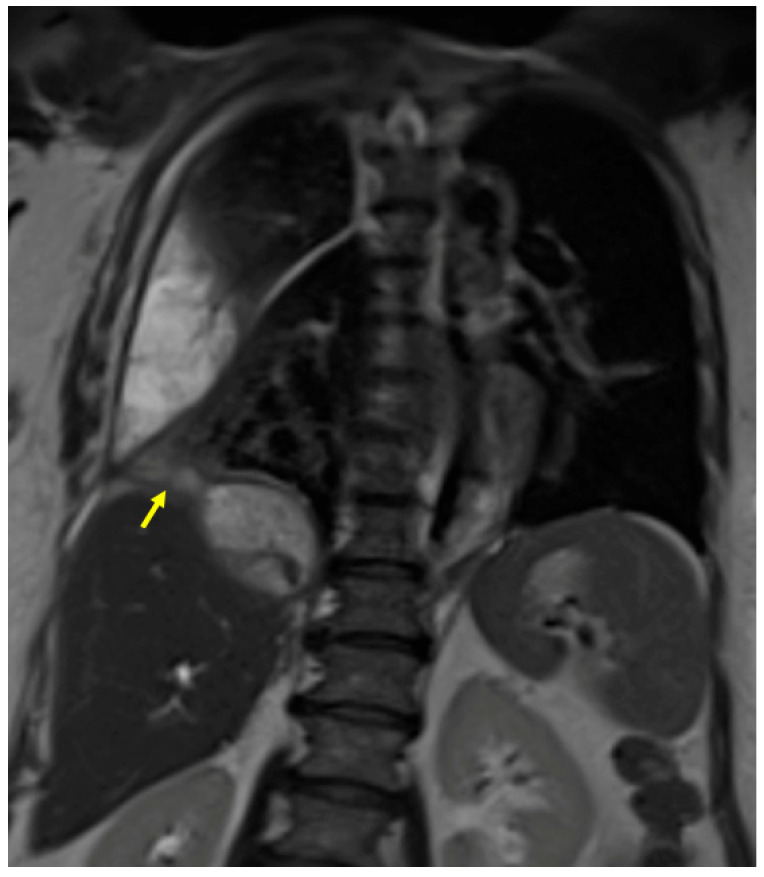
MRCP, coronal planes, and T2-weighted sequences (same patient as Figure 5). MRCP confirmed a right pleural empyema, the collection located in between the diaphragm and the liver, and the fistulous tract (arrow).
